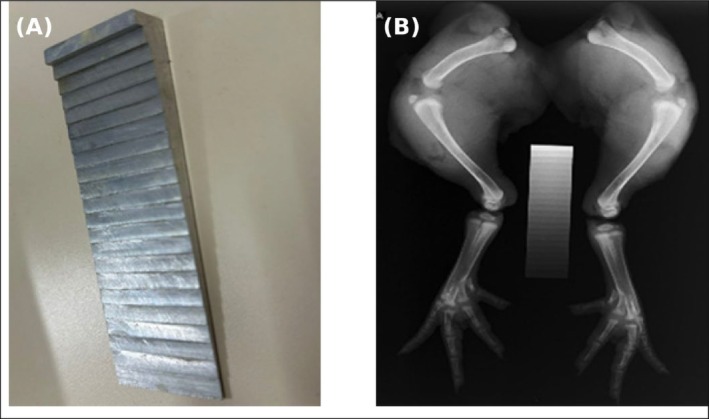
Radiographic bone densitometry of broiler chickens: (A) aluminum step wedge used for calibration; (B) radiographic image of the femur showing the evaluated regions.
